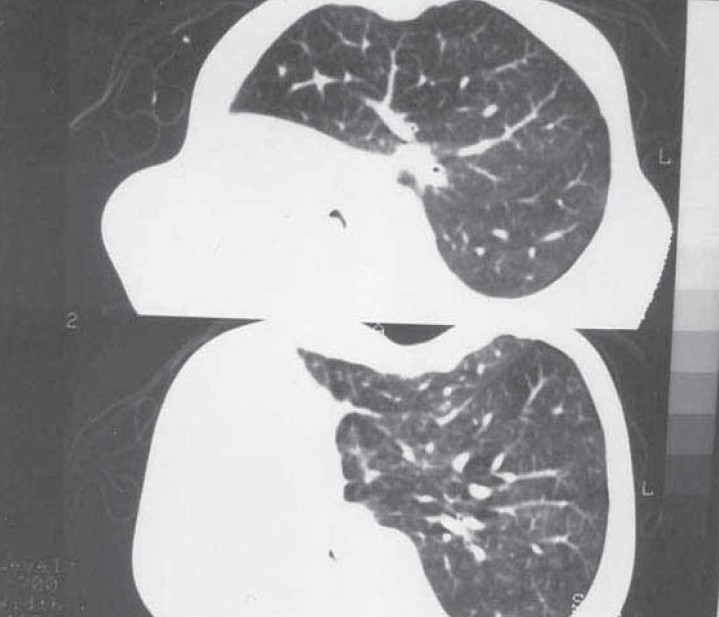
CT chest showing absent aerated lung on the right side with herniation of the left lung.
